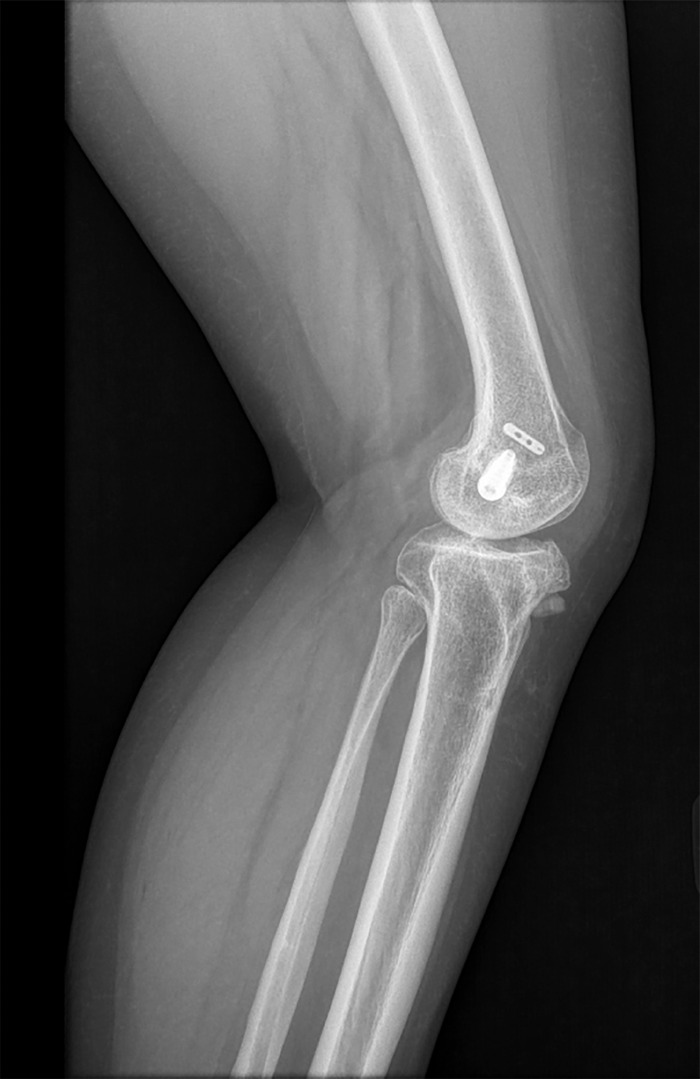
Six-week postoperative lateral radiograph of the left knee shows appropriate positioning of the tunnels and hardware.
